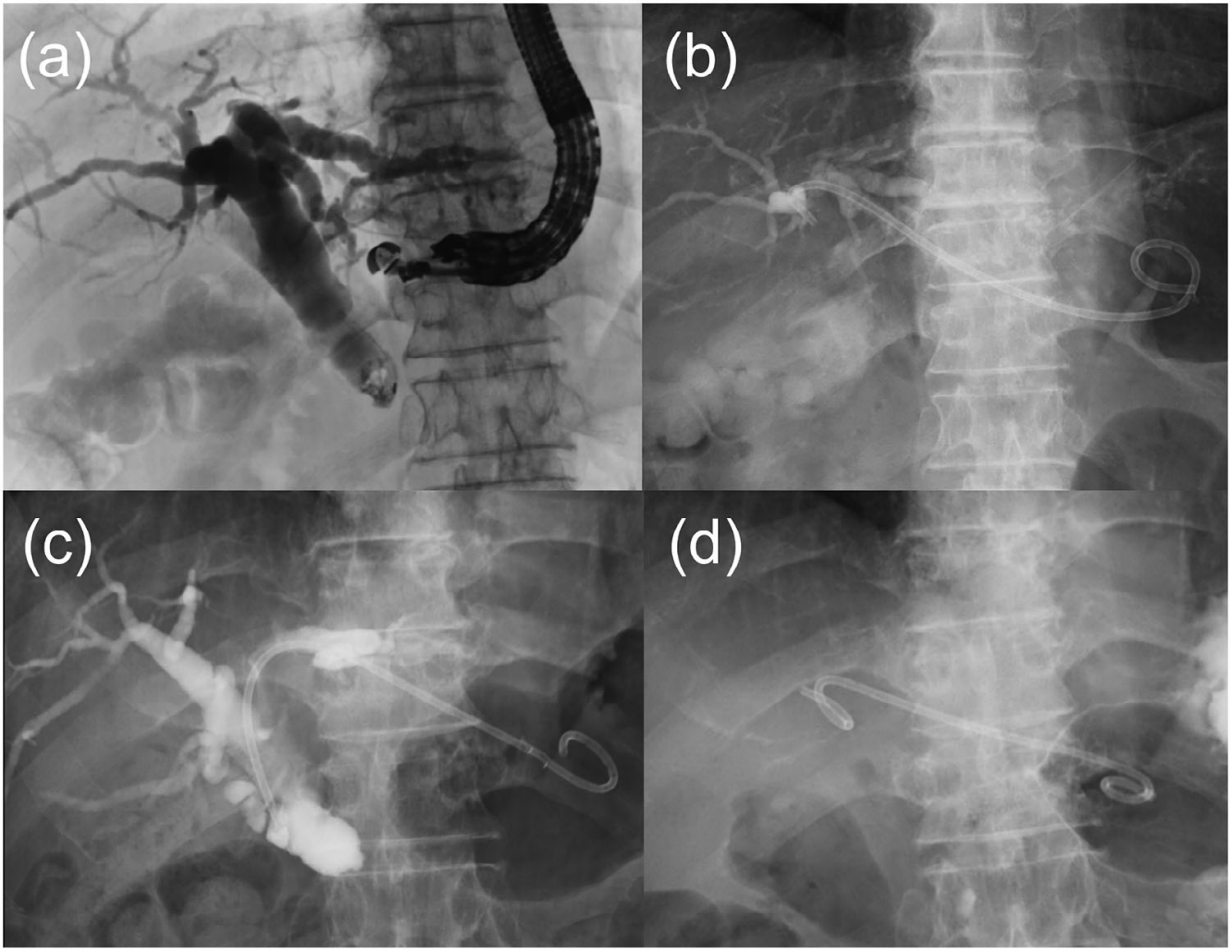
Endoscopic ultrasound‐guided hepaticogastrostomy (EUS‐HGS). (a) Fluoroscopy during EUS‐HGS. There was an obstruction in the distal bile duct, and dilatation of the bile duct upstream was observed. The area distal to the bile duct obstruction was not contrasted. (b) The plastic stent was placed via the stomach from B3. The end of the stent was located at the left hepatic duct. (c) On day 19 after EUS‐HGS, the HGS stent was exchanged. A 7‐Fr single‐pigtail plastic stent was placed with the tip positioned in the common bile duct. (d) On day 77 after EUS‐HGS, a 7‐Fr double‐pigtail plastic stent was placed for the HGS stent.
